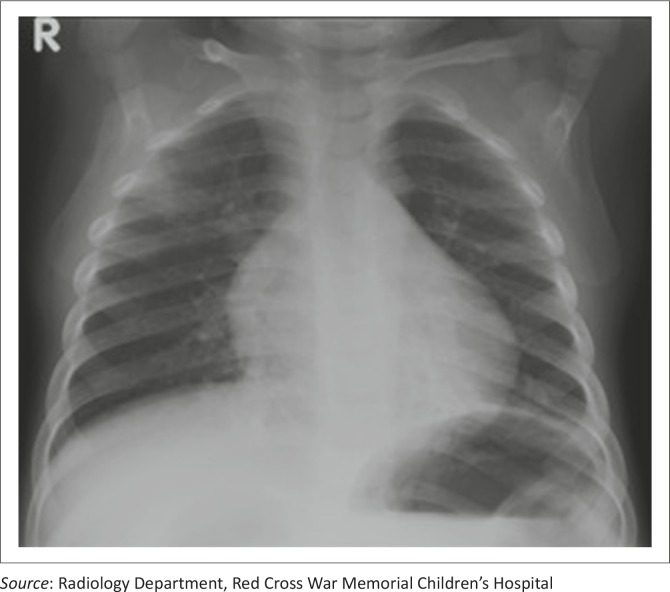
Chest radiograph on admission, with features of bilateral pneumonia.
